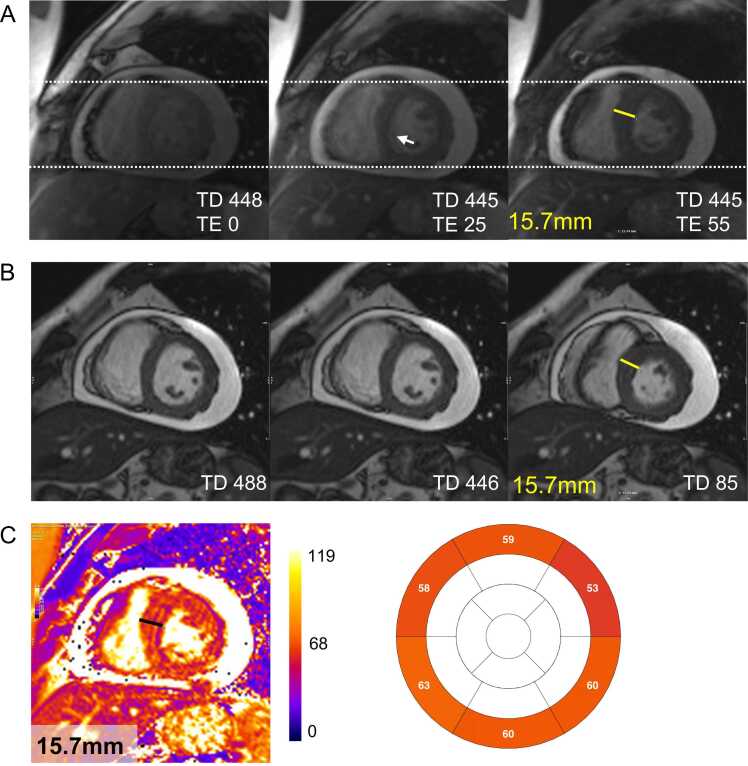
Mistriggering and residual cardiac motion. A patient with arrhythmias and a large circumferential pericardial effusion presented with heart “swinging” within the pericardial cavity. (A) T2-weighted source images erroneously acquired at different cardiac phases demonstrate noticeable variations in septal wall thickness. The trigger delay (TD) values extracted from the DICOM data may be misleading in cases of extreme arrhythmia. The white arrow in the second image highlights residual cardiac motion, resulting in blurred myocardial borders. (B) For comparison, short-axis cine images acquired at the same slice location can help estimate the approximate cardiac phase in which the individual source images were obtained. (C) Myocardial T2 values in the final map likely represent a mixture of different tissues, such as blood, myocardium, and pericardial effusion. The map should not be considered for clinical interpretation. *DICOM* digital imaging and communications in medicine, *TE* echo time
